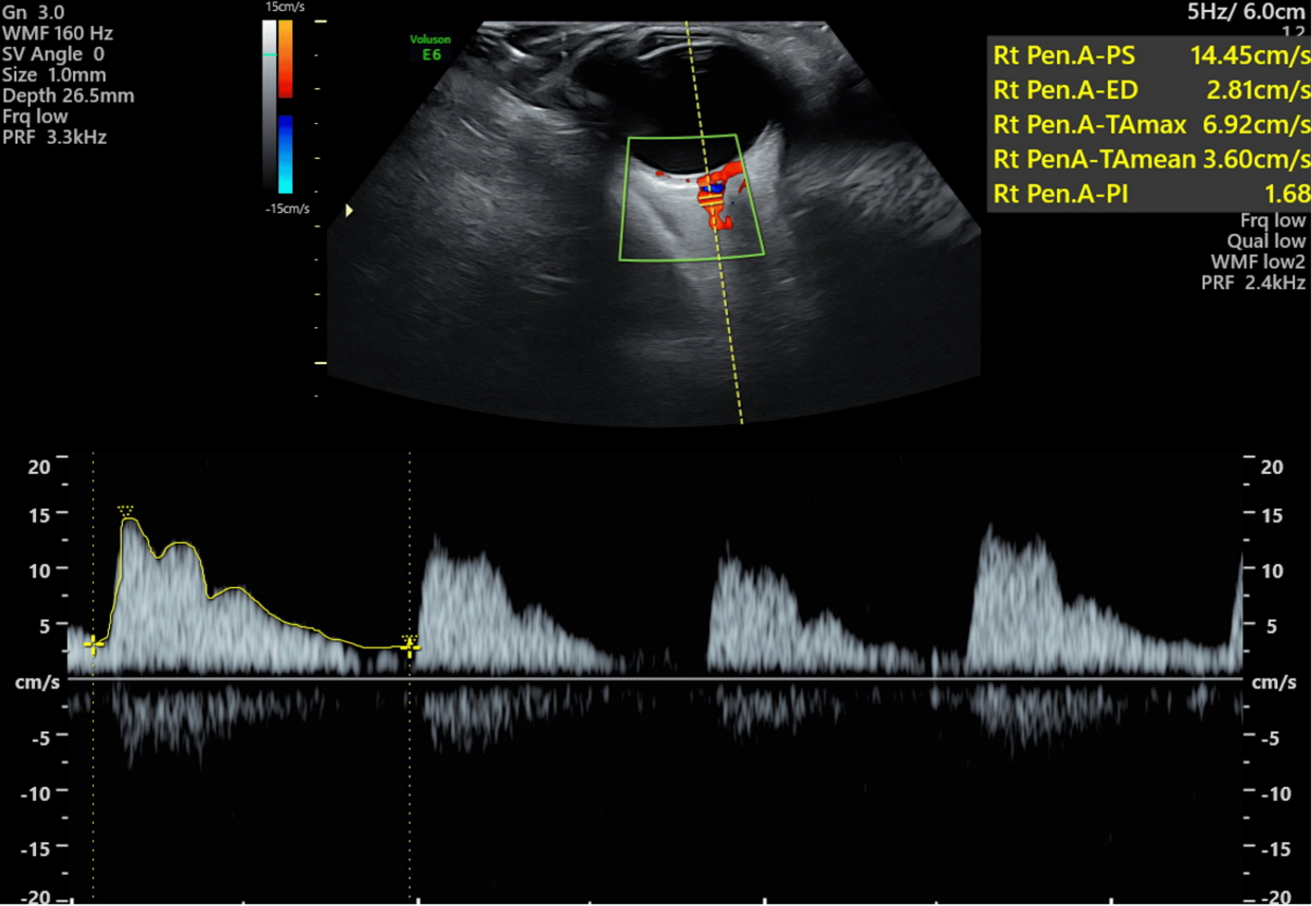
Doppler imaging of the ophthalmic artery showing mean enveloped velocity and Pulsatility Index (PI).
